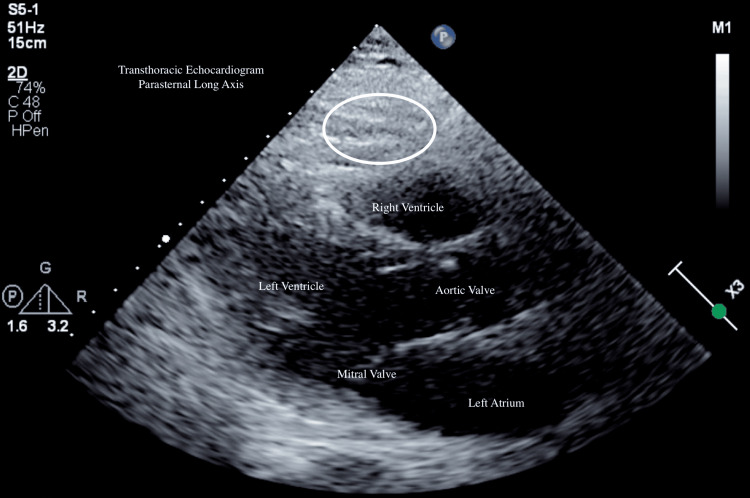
Interval transthoracic echocardiogram (PLAX) PLAX: Parasternal Long Axis View The white circle shows the resolved pericardial effusion.
